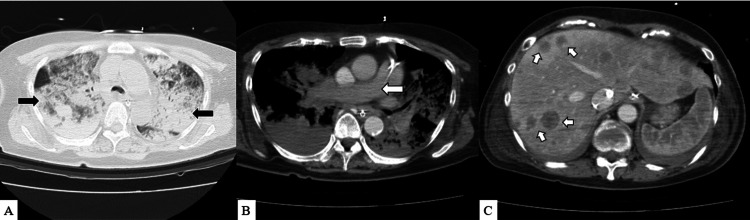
CT findings on the eighth day (A) Black arrow: severe lung field consolidation is observed. (B) White arrow: no contrast defect of the pulmonary artery is observed. (C) White arrow: multiple masses are observed in the liver CT: computed tomography
